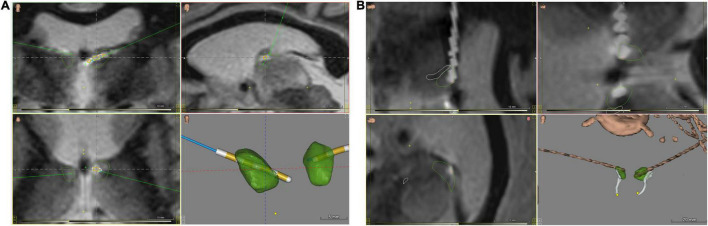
ANT nuclei images with brain atlas overlay of postoperative CT/MRI merge, visualizing implanted RNS depth electrodes. (A) Coronal (upper left and lower left) and sagittal (upper right) views, and 3D-rendered image of implanted ANT nuclei without background brain. (B) Close-up views of implanted leads from rotated sagittal (upper and lower left) and oblique (upper right) views, along with an oblique view 3D-rendered image of implanted ANT nuclei without background brain. ANT nuclei marked in green, mammillothalamic tracts in white, electrodes in brown. Images generated with WayPoint Navigator version 4.6.6.
